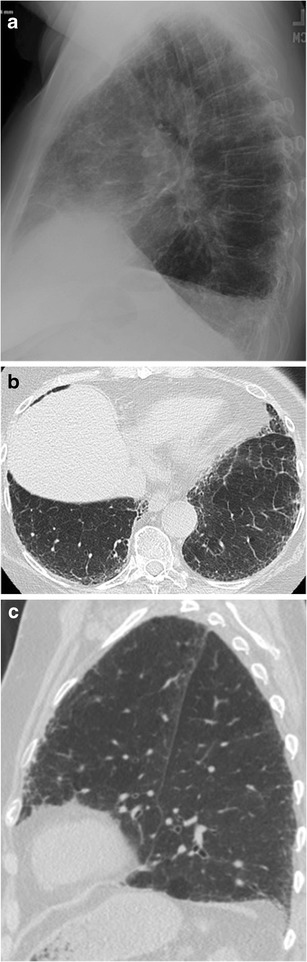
84-year-old woman with idiopathic pulmonary fibrosis. On the lateral radiograph (a), reticular opacities are easily seen through an opaque retrosternal space. Although the retrocardiac space is more lucent, reticular opacities are not seen at the lung bases, despite the basilar predominance typical of UIP demonstrated on axial (b) and sagittal (c) CT slices
